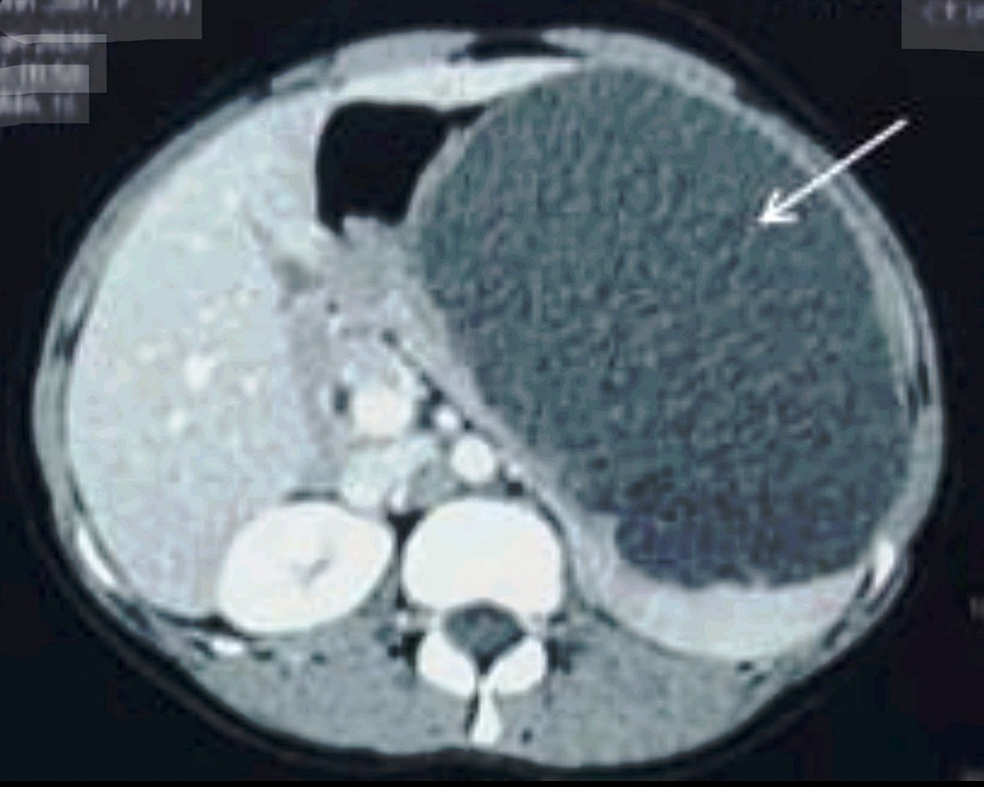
CT abdomen showing a large cystic lesion ( white arrow ) of spleen
